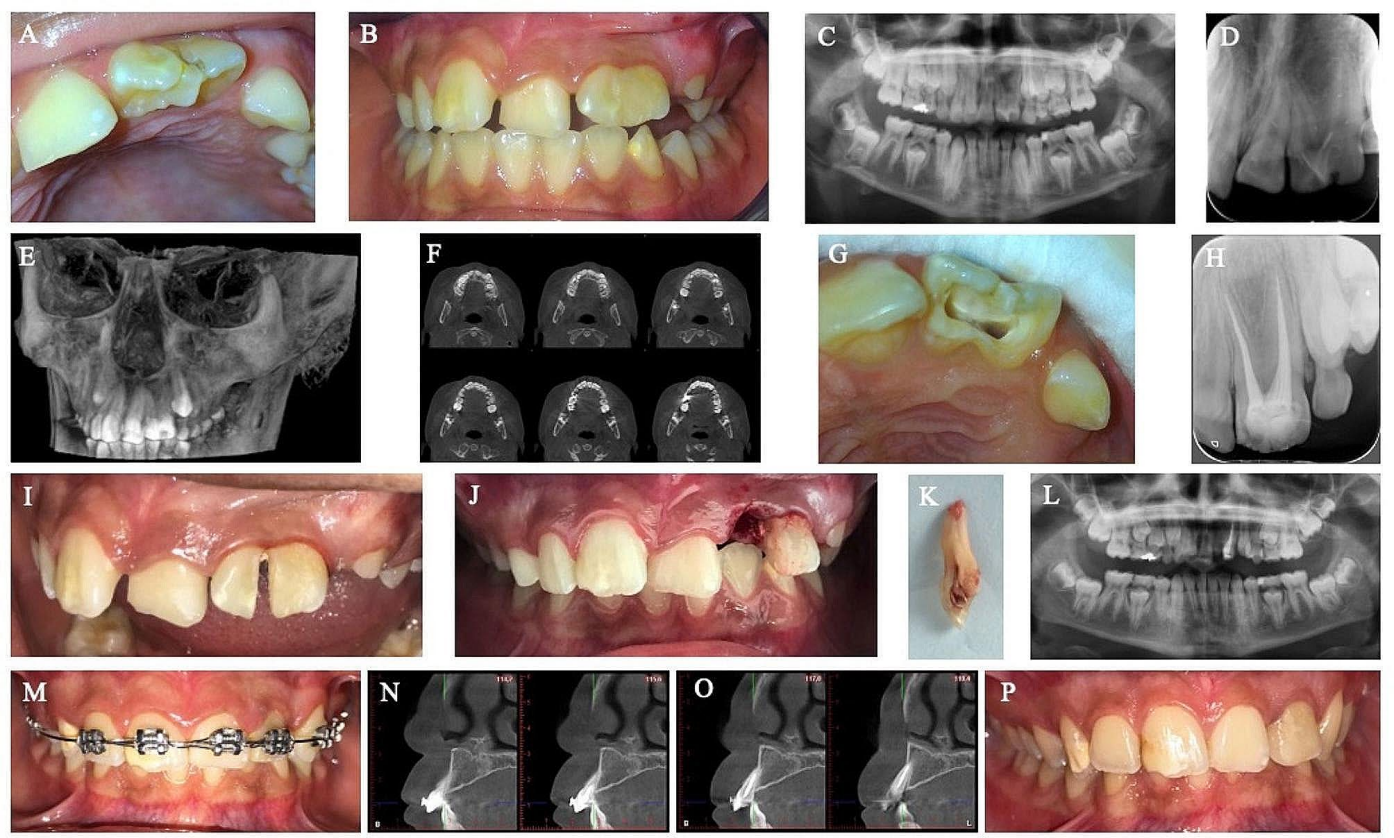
Treatment progress of the 9-year-old girl with a concrescent left lateral incisor. (A–D) pretreatment intra-oral photograph and panoramic and periapical radiographs, (E–F) 3-dimensional reconstructed images and coronal views of CBCT, (G–H) the root canal treatment of double incisor, (I–K) the hemisection procedure and extracted tooth, (L–M) panoramic radiograph and orthodontic alignment after the hemisection, (N–O) the axial CBCT images 18 months after hemisection, (P) the intraoral photograph six years after hemisection
